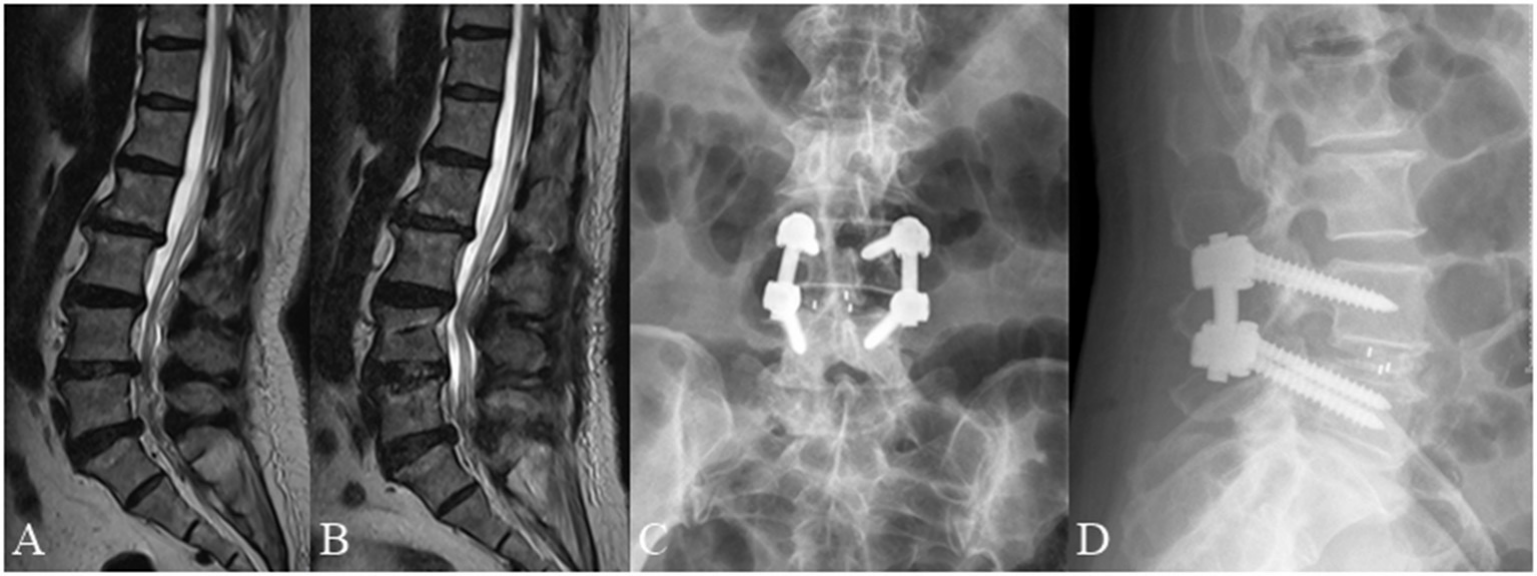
A 66 year old female complained of low back pain for 3 months underwent posterior lumbar decompression and instrumented interbody fusion at L4/5. (A) Pre-operative lumbar MRI showed that the nerve roots were redundant above the L4/5 intervertebral disc. (B) Post-operative lumbar MRI obtained 3 days after surgery demonstrated significant relief of RNRs. (C,D) Lumbar radiographs obtained 3 months after surgery demonstrate internal fixation in good position without cage subsidence or migration.
